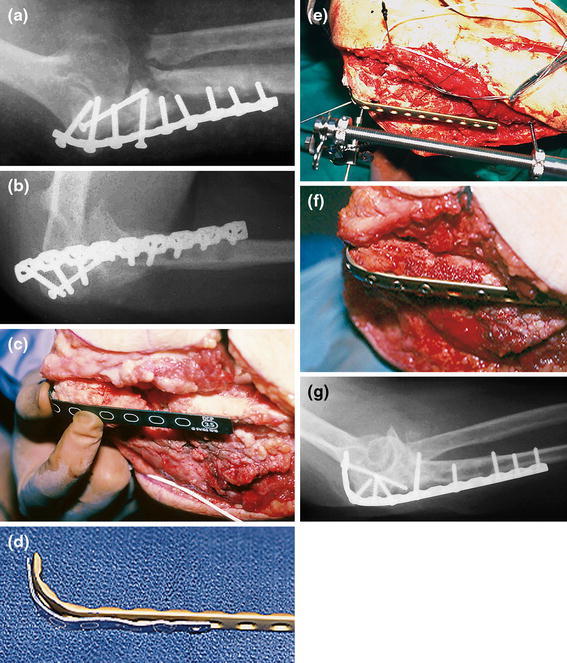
Nonunion after internal fixation of a posterior Monteggia fracture dislocation. a, b Loosening of the plate and screws and instability; c, d After hardware removal, debridement, a posterior plate is contoured to cradle the proximal ulna; e Using the femoral distractor alignment was obtained; f Final appearance intra-operatively after plating and bone grafting; g Postoperative radiograph. Case courtesy Jesse B. Jupiter (*Source*: Marti and Kloen [26]) (Copyright owned by David Ring, MD, PhD)
